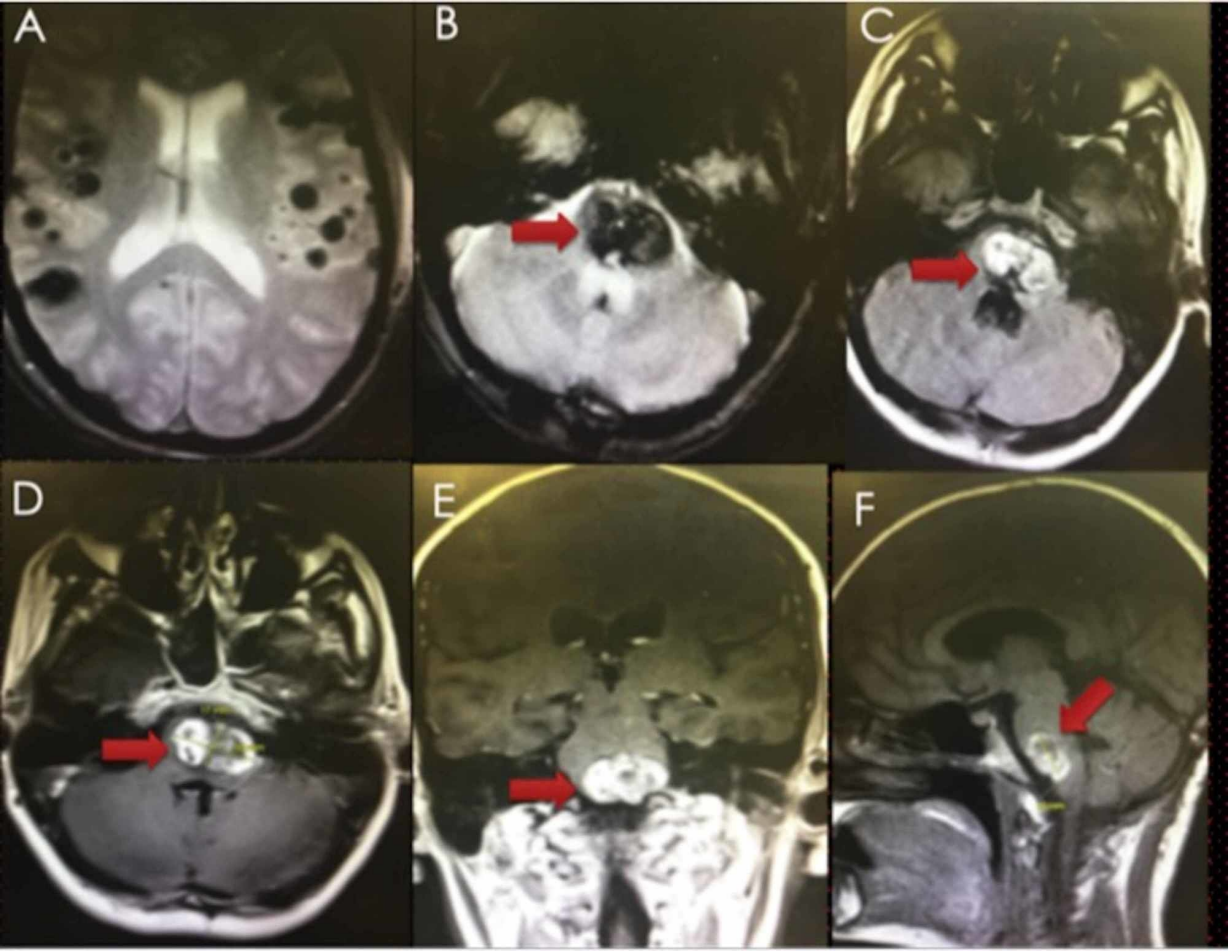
Preoperative MRI (GRE (A&B), T1 FLAIR (C), and T1W gadolinium contrast images (D-F)) showing multiple intracranial cavernomas (supratentorial A, pontomedullary B-F, red arrows). MRI: magnetic resonance imaging; FLAIR: fluid-attenuated inversion recovery
